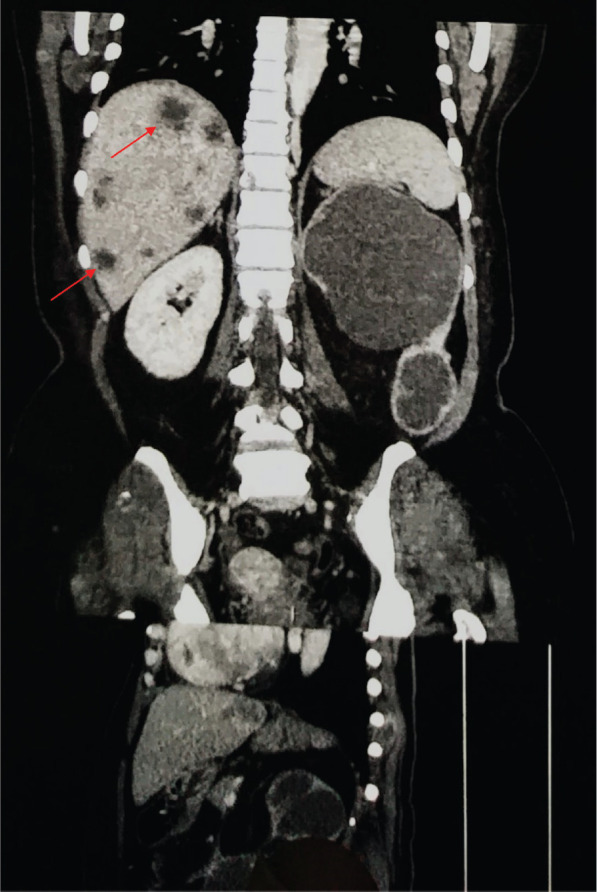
Contrast-enhanced CT scan of the abdomen and pelvis (Coronal section) representing metastasis (red arrows).
